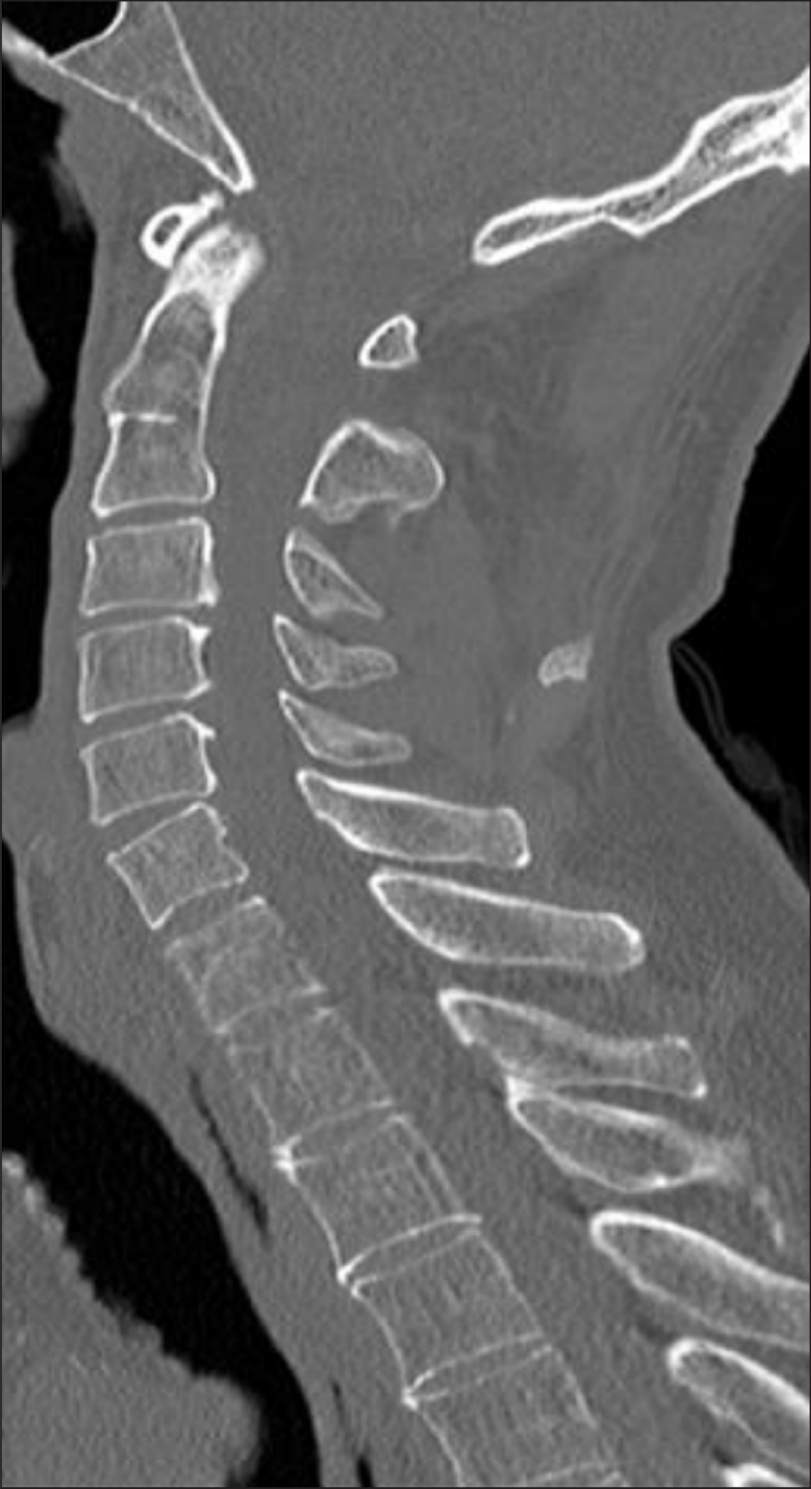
Therefore, we can conclude that the dense intravertebral images on the contrast-enhanced CT imaging resulted well from contrast accumulation in intravertebral venous collaterals [2].
